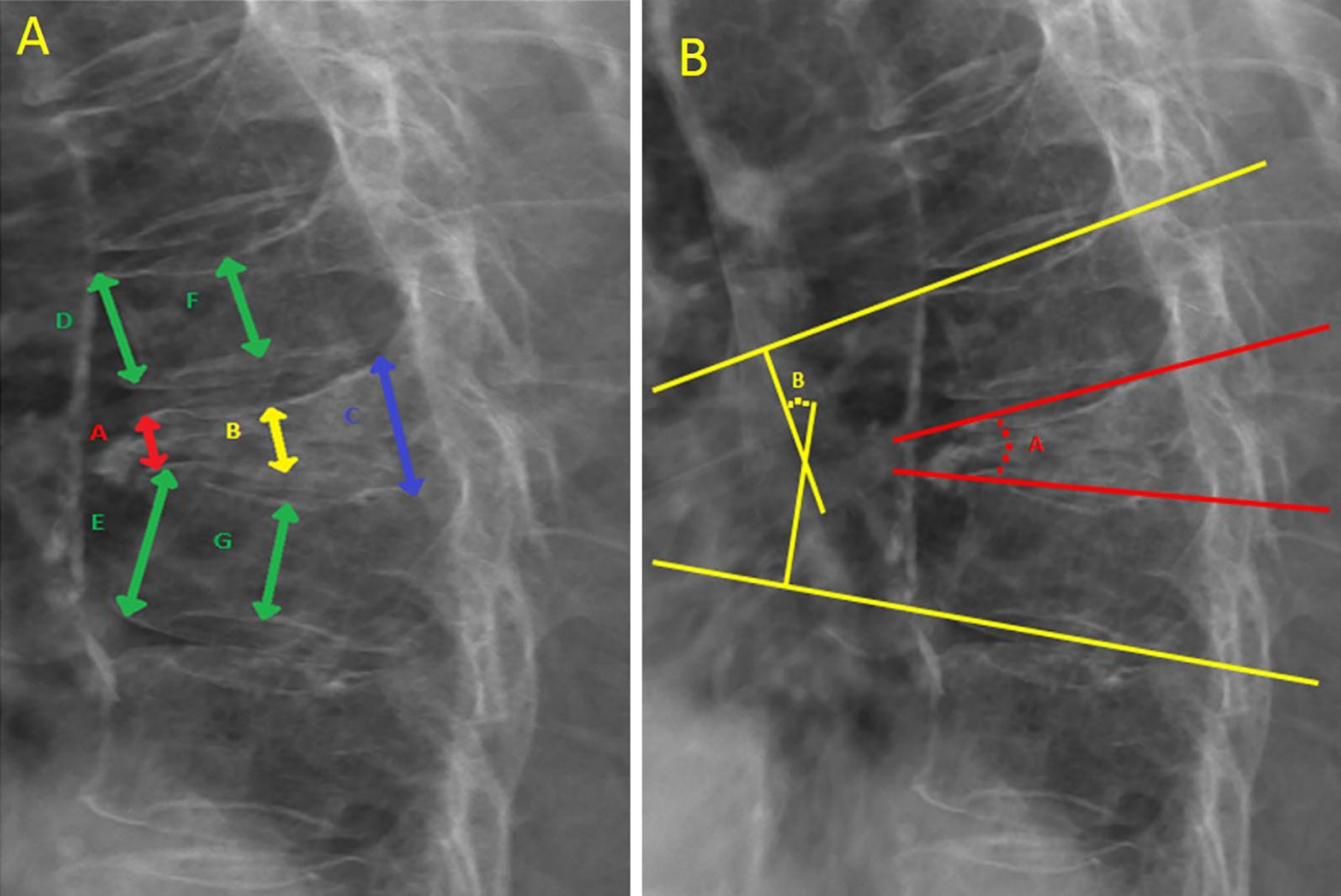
Demonstrated radiographic parameters. (A) Evaluation of the severity of compression fracture. A: anterior vertebral height, B: middle vertebral height, C: posterior vertebral height, D: superior anterior vertebral height, E: inferior anterior vertebral height, F: superior middle vertebral height, G: inferior middle vertebral height. (B) Measurement of sagittal angle of the fractured vertebrae. A: local kyphotic angle, B: Cobb angle.
